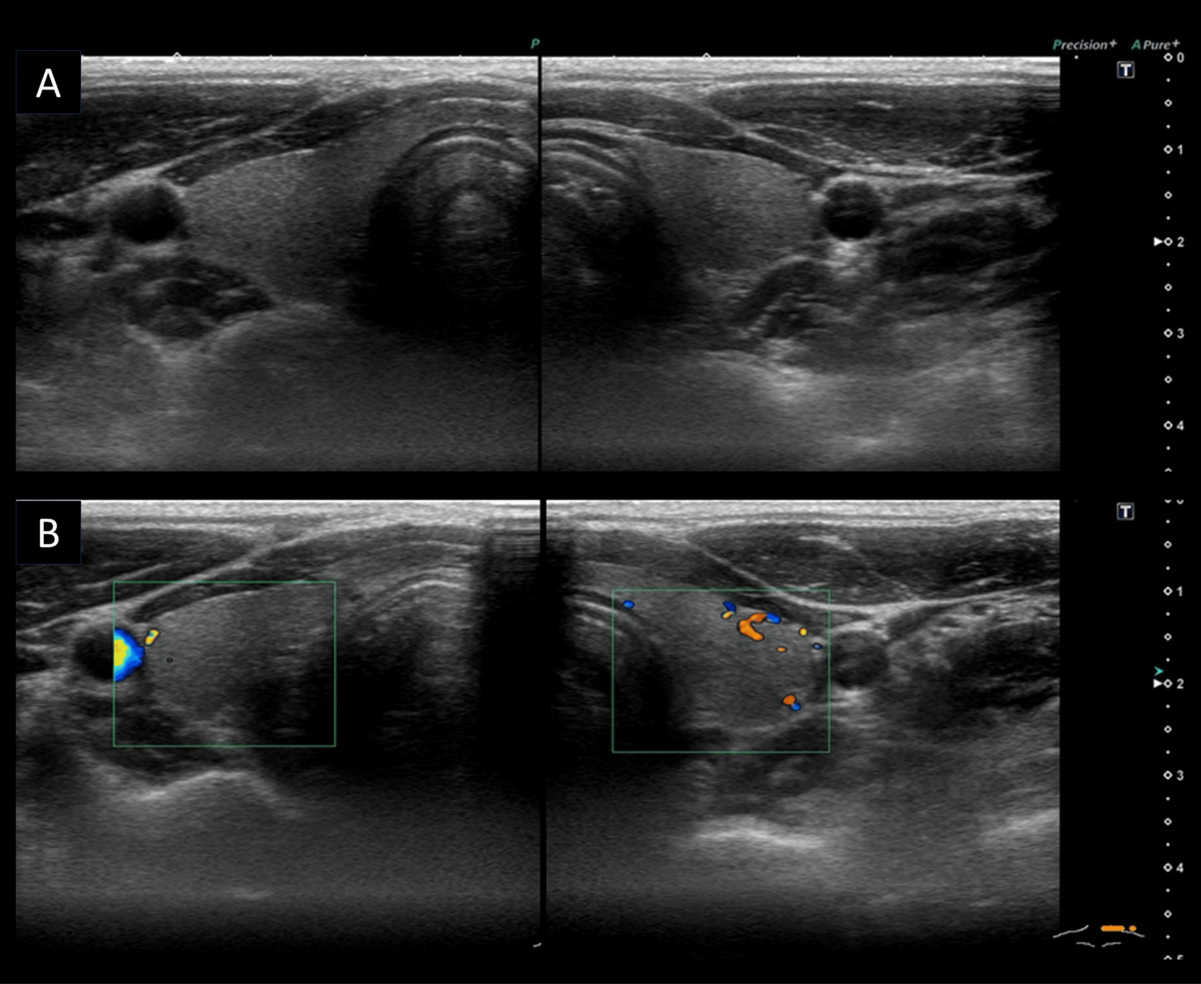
Ultrasound findings of the proband (A) B-mode and (B) color Doppler images showing no abnormal findings in either lobe of the thyroid.
